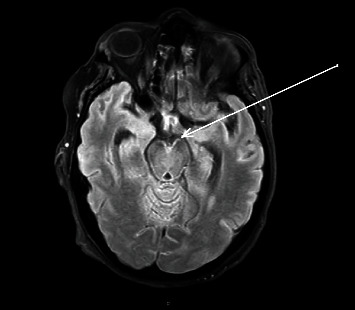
Brain MRI showing linear hyperintensities in basal segments and VIII, VII, V, and III cranial nerves (FLAIR sequence) (please see the arrow).
